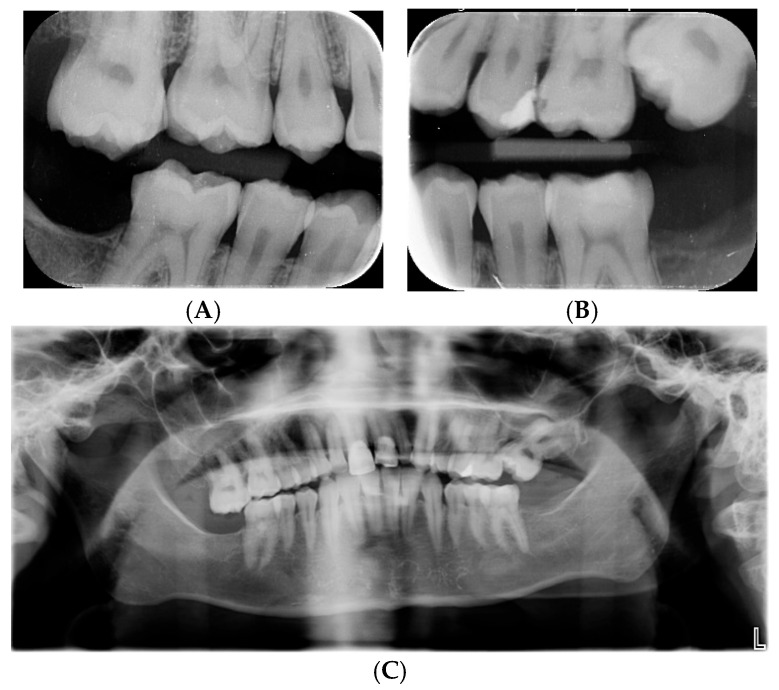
(A,B) Bitewings radiograph at the age of 18 years shows caries 26 and ectopic 27, yet no resorption of 26. Low contrast between enamel and dentin is observed. (C) Panoramic radiograph at the age of 28 years shows movement artifact as well as an ectopic 27 causing resorption distally on 26. Teeth 18 and 28 are congenitally absent, but 37, 38, 47, and 48 were removed at the age of 20 due to ectopic positioning and resorption.
